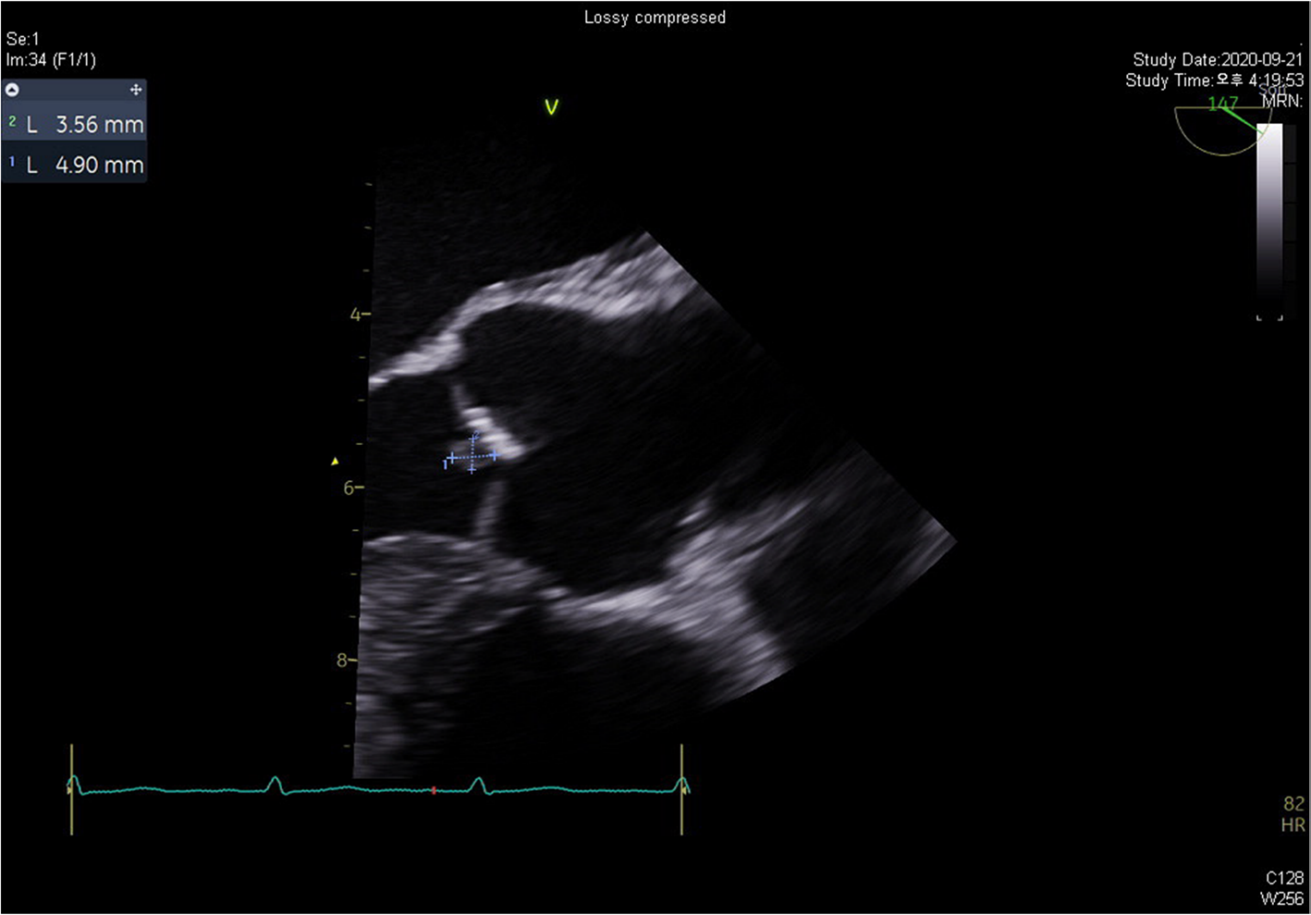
Transesophageal echocardiography image shows aortic valve vegetation
